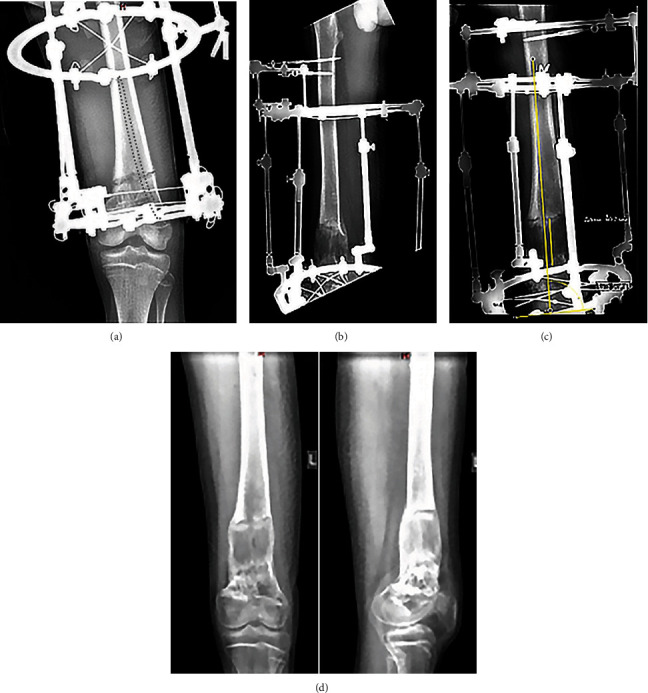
Radiographs of the left femur: (a) on the day of the intervention (position of the implant is not visible on radiographs; eventual position is presented with dashed grey line); (b) after 30 days of distraction; (c) at the end of distraction; (d) on the frame removal.
